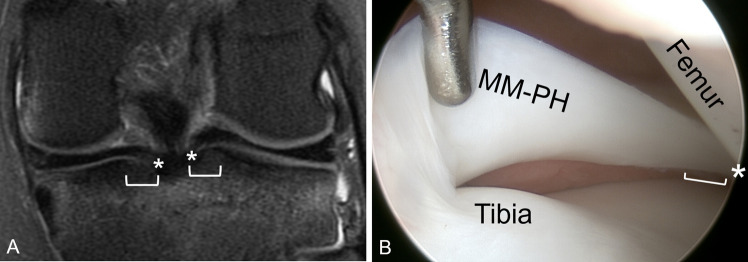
Coronal proton-density-weighted fat-suppressed MRI from a 22-year-old man (A) with ACL tear and arthroscopic view from a 12-year-old boy (B) show a normal posterior root of the medial meniscus which includes not only the entheseal attachment (*), but also the adjacent meniscus within 1 cm (brackets), continuous with the medial meniscus posterior horn (MM-PH). The entheseal attachment is a difficult region for the arthroscopist to visualize directly. Arthroscopic view courtesy of Theodore J. Ganley, MD
